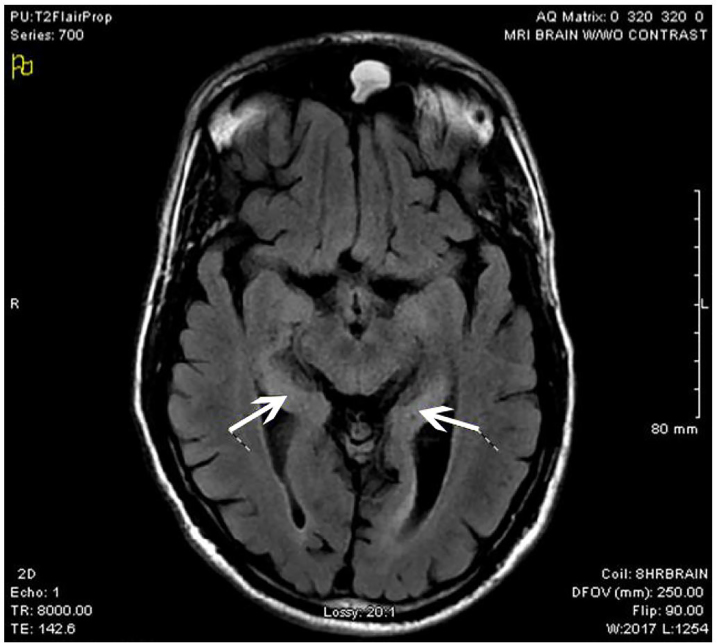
Axial T2 FLAIR sequence magnetic resonance imaging showing enhancement of the medial temporal lobe (arrows). *FLAIR,* fluid attenuated inversion recovery
